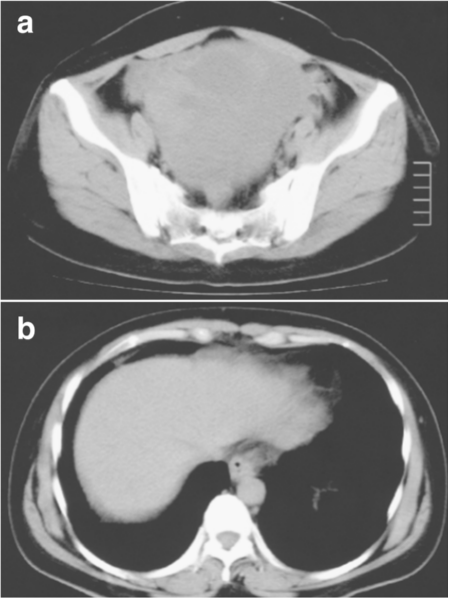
Computed tomography demonstrated a large, round mass with a maximum diameter of 15 cm in the pelvic cavity (a) and no acites or pleural effusion (b)﻿
